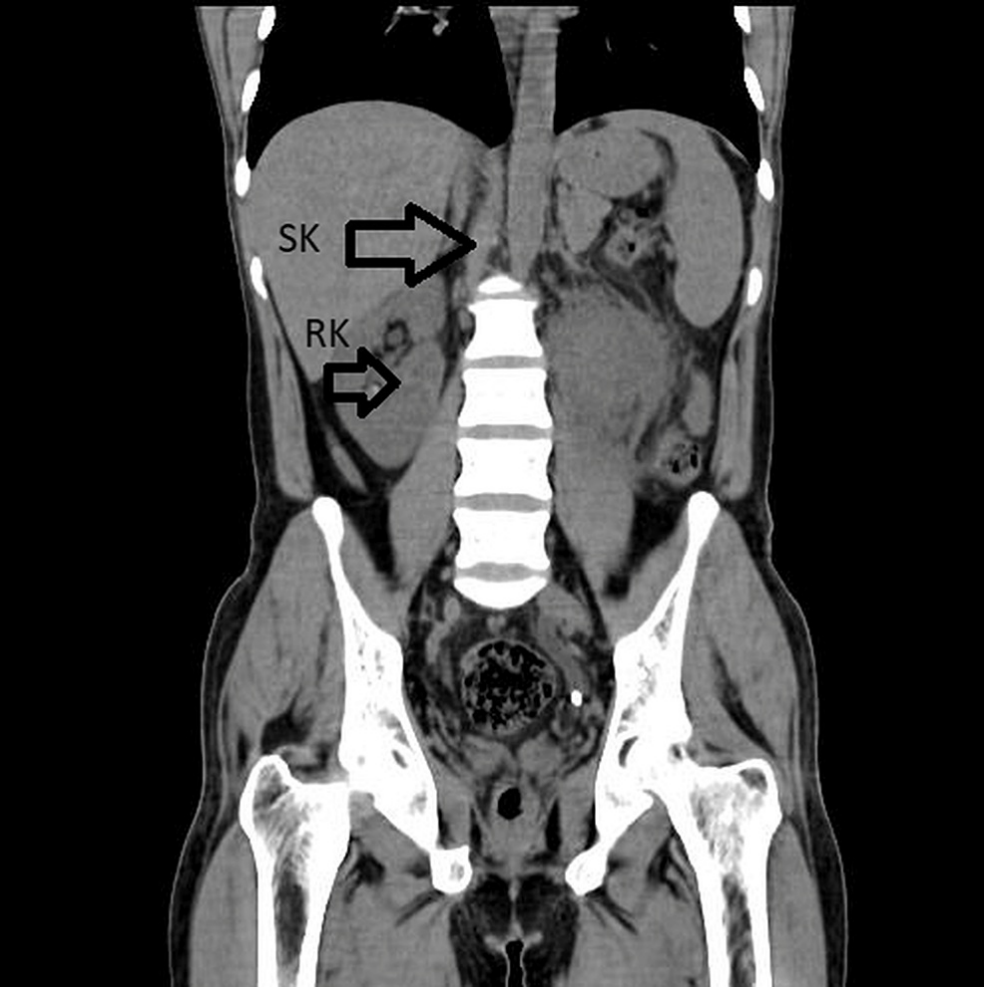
Abdominal CT scan coronal image of the abdomen showing the supernumerary kidney and the hydronephrotic right kidney
